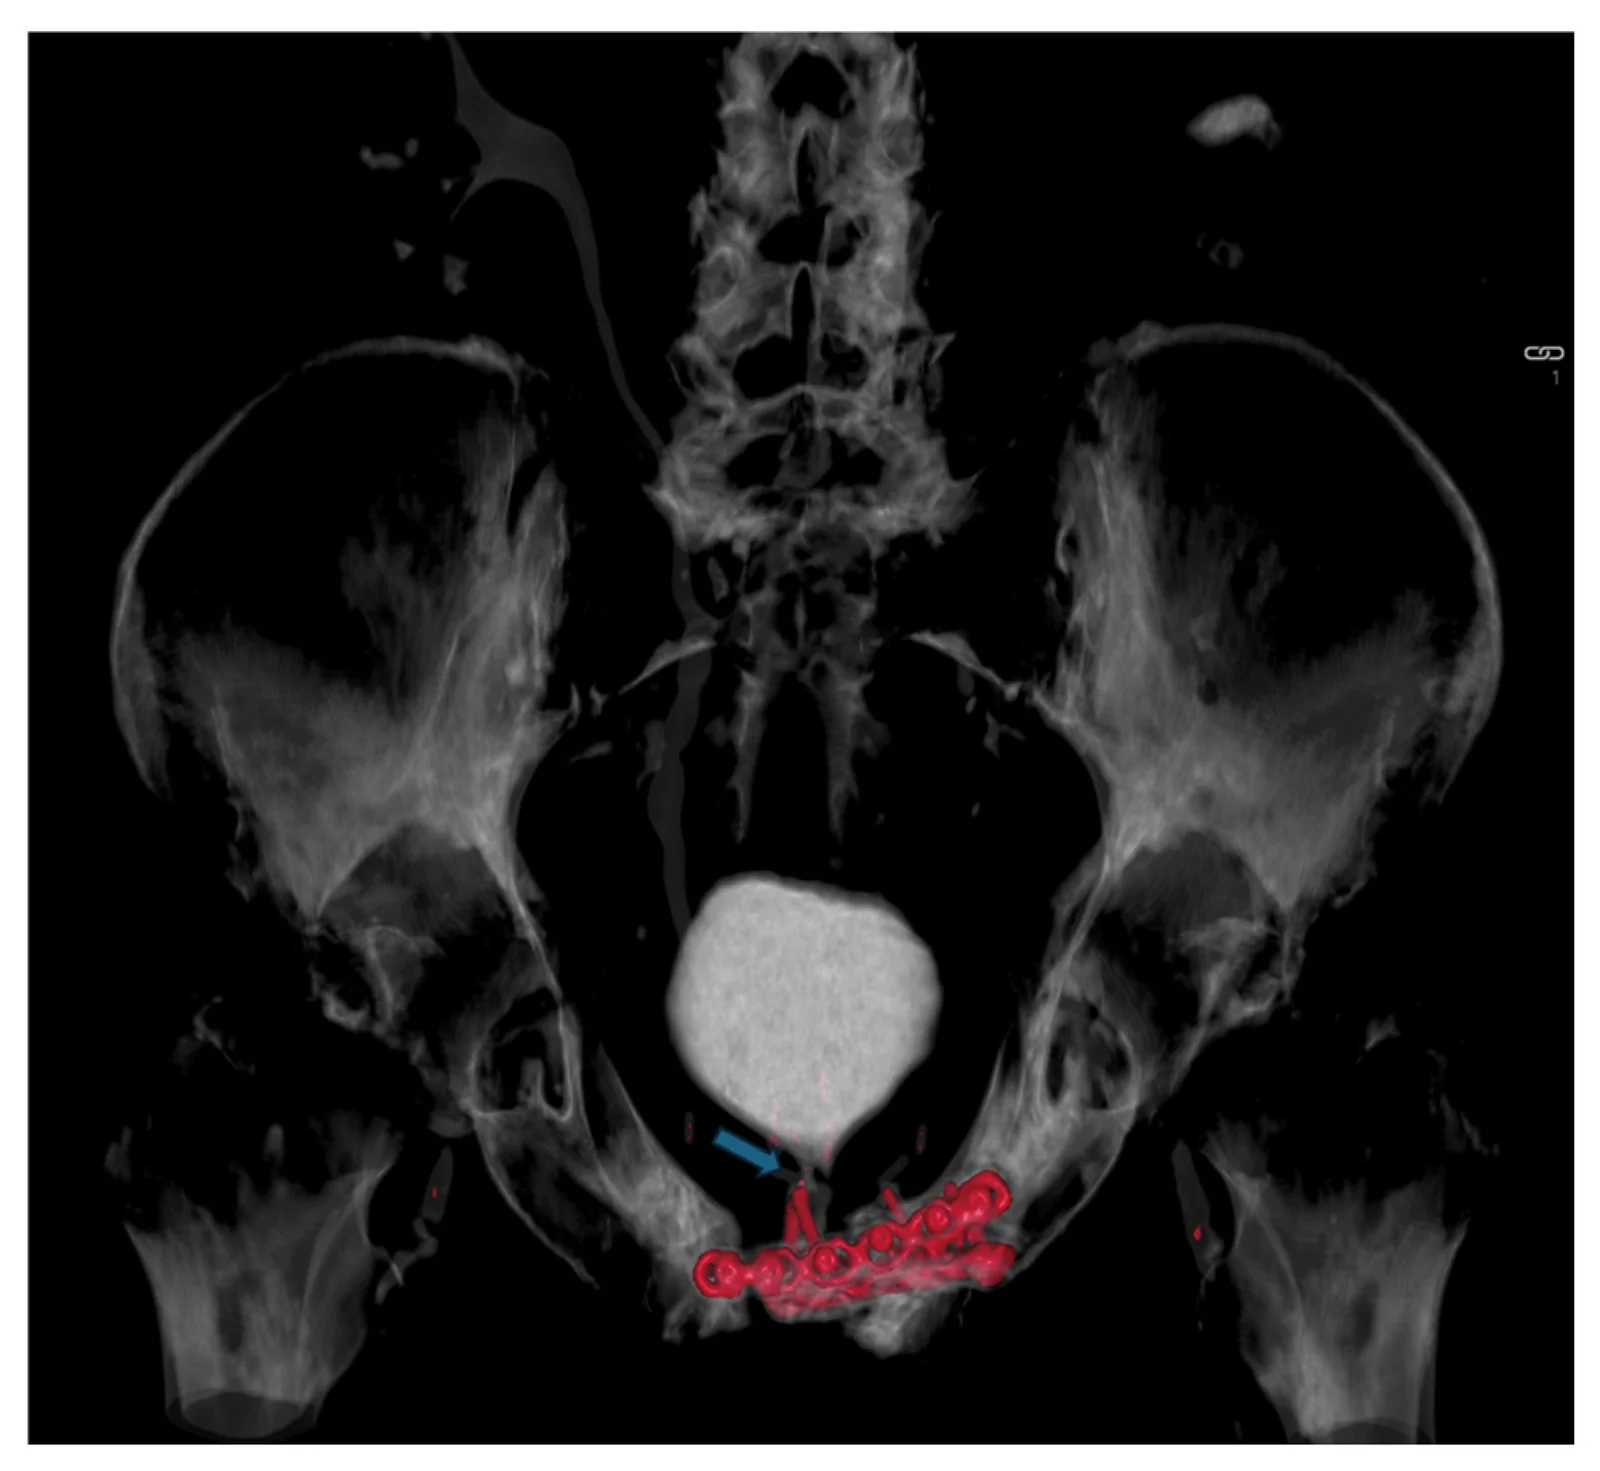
Excretory-phase CT of the abdomen and pelvis with volume-rendered technique (VRT), bone, and metal reconstructions demonstrating orthopedic osteosynthesis screws from previous pelvic fracture fixation (highlighted in red) projecting beyond the pubic bone into the adjacent pelvic soft tissues (frontal plane). The screw tips are surrounded by contrast-opacified urine at the level of the urethra (arrow), demonstrating their close relationship to the urethral lumen.
